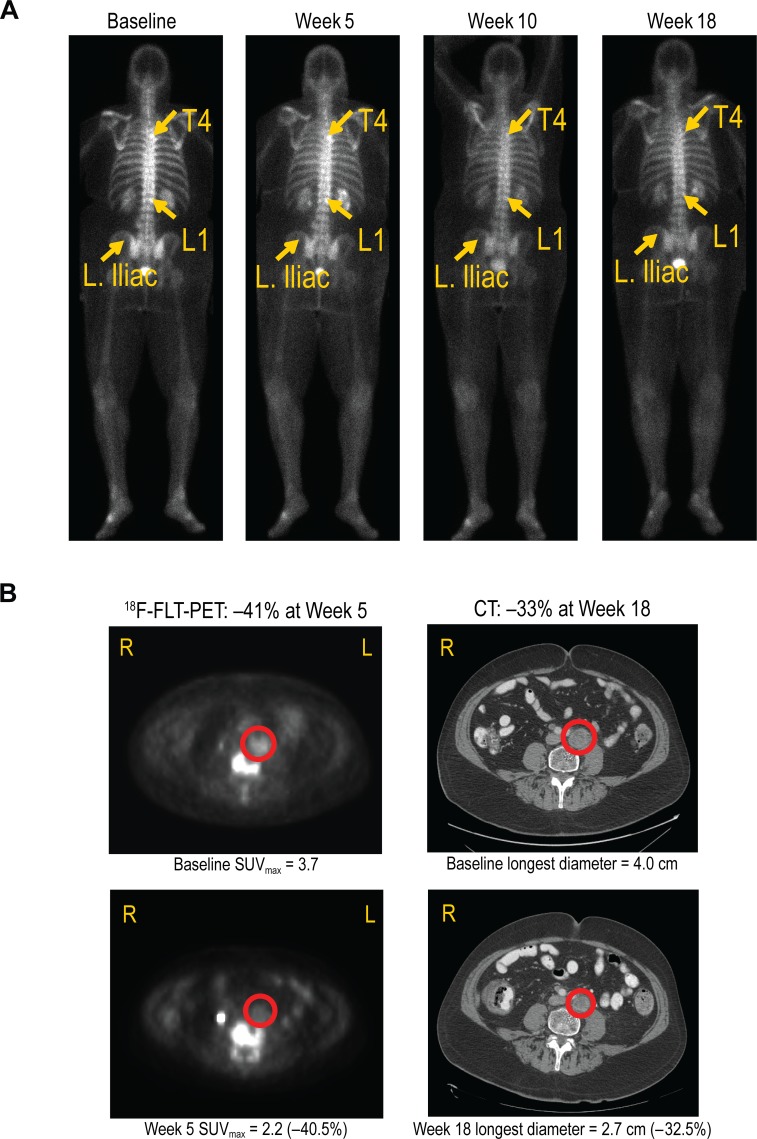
A. Complete response in a 66-year-old patient with prostate cancer treated with 300 mg AMG 208 (bone scans). At baseline, bone metastasis was present at T4 and L1. At week 18, evidence of bone metastasis was not observed. B. Partial response in a 63-year-old patient with prostate cancer treated with 400 mg AMG 208 (^18^F-FLT-PET and CT scans).
